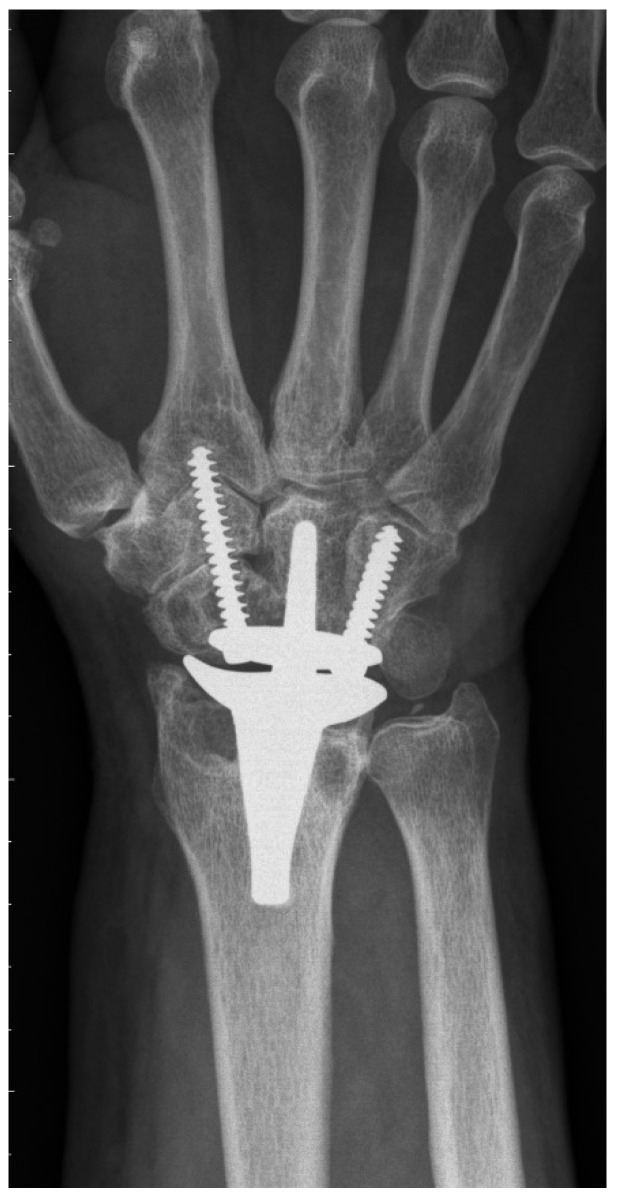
Final radiograph (72 months postoperatively) of a CCR cohort patient with massive radial impaction syndrome.
